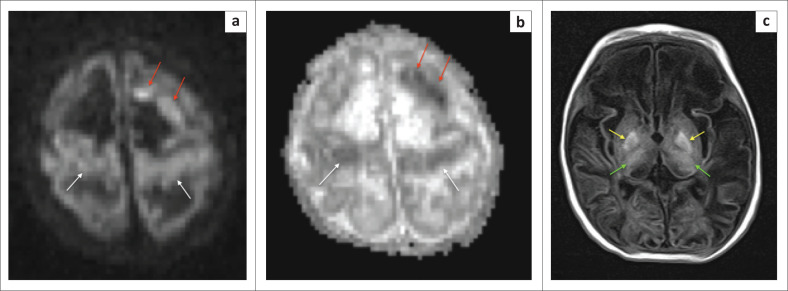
A 15-day-old female child born after abruptio placentae with poor Apgar scores of 0/10 and 1/10 at 1 and 5 min, respectively. Head and body cooling were instituted but the clinical condition remained poor with seizures. The diffusion sequence (a) shows resolving diffusion signal abnormality (white arrows) at the perirolandic cortex and paracentral lobules bilaterally but there is persistent restricted diffusion at the left frontal lobe (red arrows) with ADC shortening in (b) because of watershed zone ischemia. (c) Note the established ischemic injury of the thalami (green arrows) and lentiform nuclei (yellow arrows) with T1-weighted hyperintensity. This is in keeping with evolving mixed subtype of hypoxic ischemic brain injury in the early neonate.
